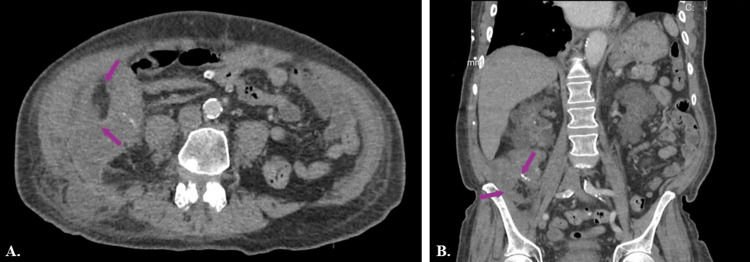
CT of abdomen and pelvis (A) transverse and (B) coronal views Purple arrows indicate right Inflammatory process noted in the right lower abdominal quadrant potentially remnant of the appendix
